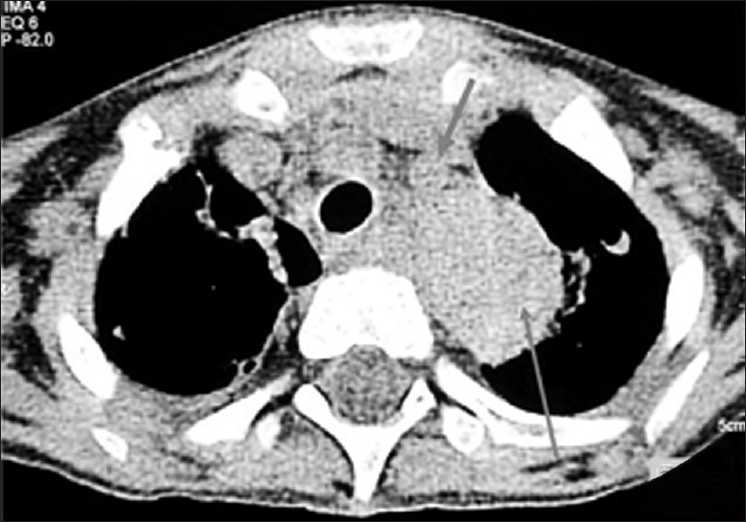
HRCT chest (mediastinal window) showing left apical mass (long arrow) at a lower level with engulfment of left subclavian artery (thick, short arrow)
